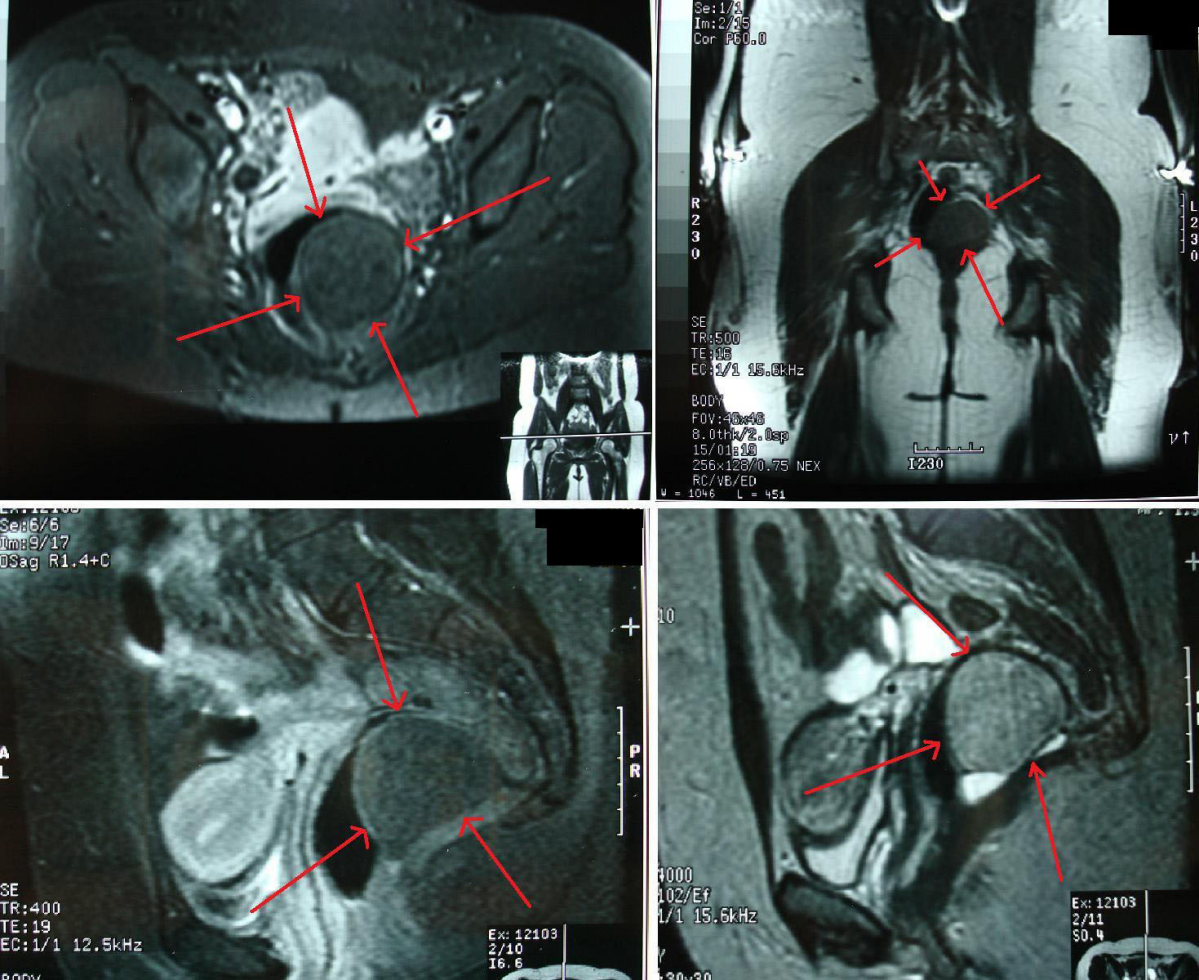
Magnetic resonance imaging scans of the axial, coronal and midline saggital areas show a 6 × 5 × 4.5 cm mass of heterogeneous signal intensity, located anterior to the distal sacrum and coccyx (arrows indicate the tumor).
